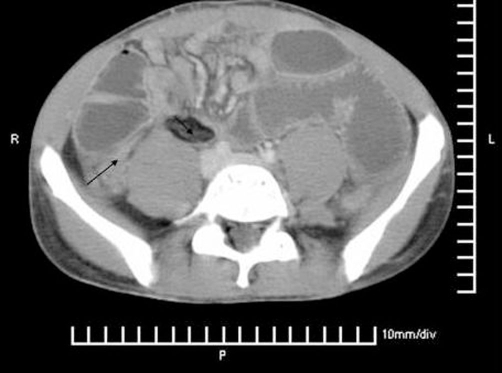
Preoperative CT scan showing acute intestinal obstruction with small bowel dilatation (arrow points towards the mass caused by the inflammation of the appendices epiploic).
